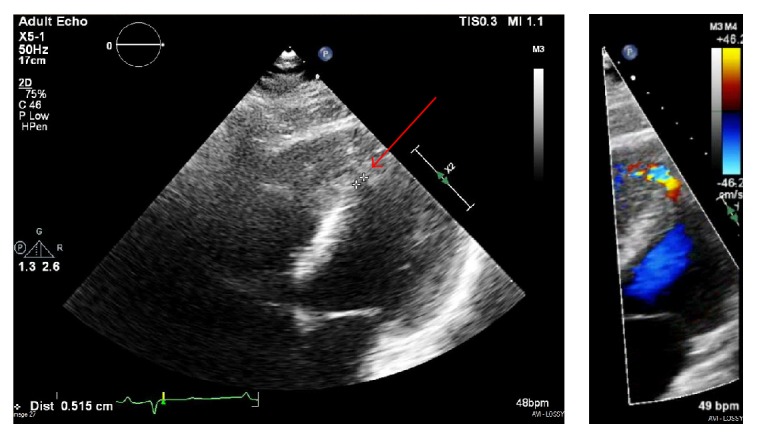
Transthoracic echocardiogram. Small, 5 mm ventricular septal defect in the distal inferoseptal/apical septum (red arrow).
